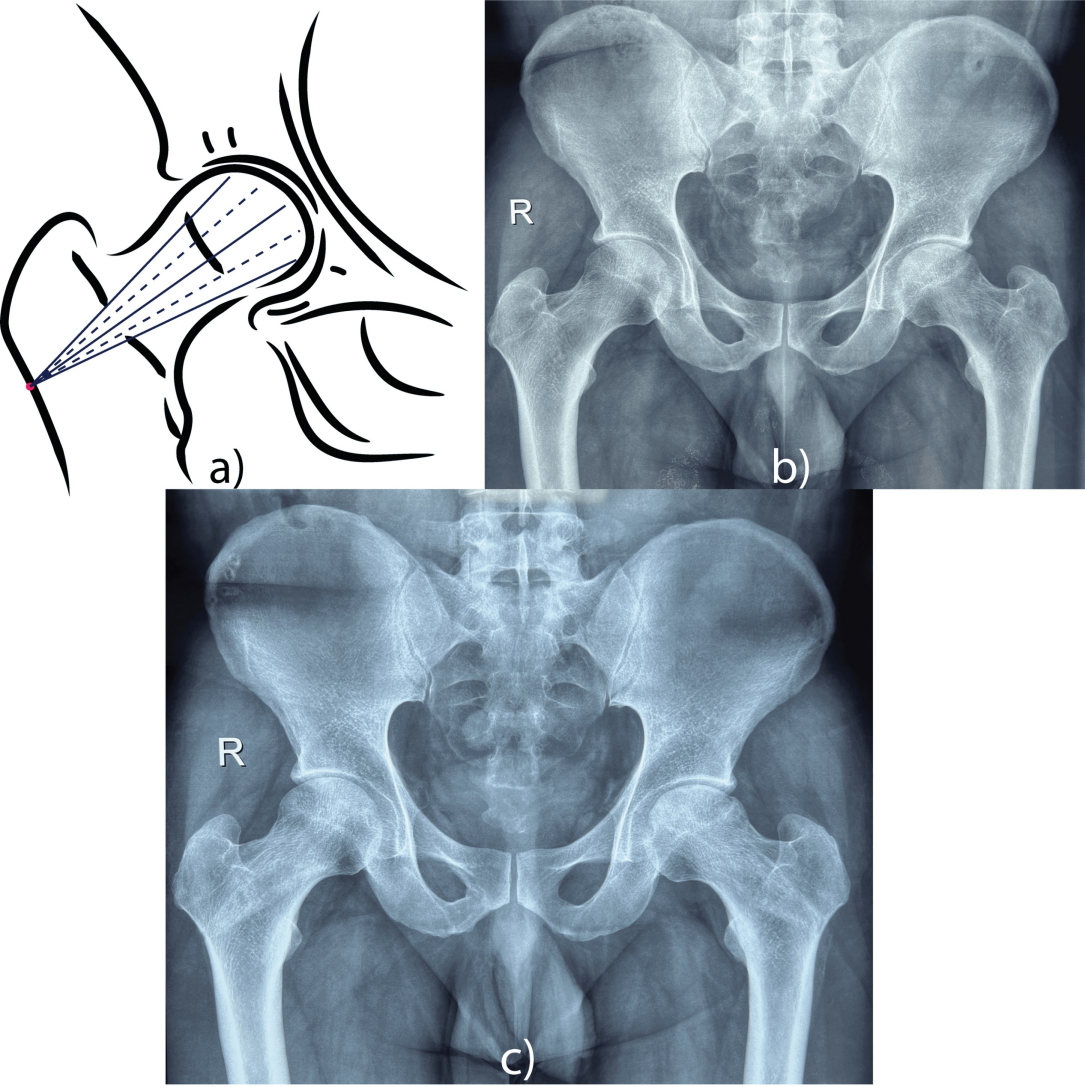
A sequential depiction of the modified core decompression technique used for treating avascular necrosis (AVN) of the femoral head, highlighting both the method and the progressive healing stages. (a) Outlines the surgical method, where a single pilot hole was drilled using a 4.5 mm drill bit into all four quadrants and the center of the femoral head through reverse drilling, following which bone marrow concentrate was inserted into the affected area. The remaining portion of the image contains radiographic images showing the stages of the disease and the healing process. (b) Stage I and Stage II AVN on the right and left sides, respectively, with a sclerotic cavity in the femoral head. (c) Complete healing of the lesion within six months, indicating the effectiveness of the combined surgical and biological approach in restoring femoral head integrity and function.
